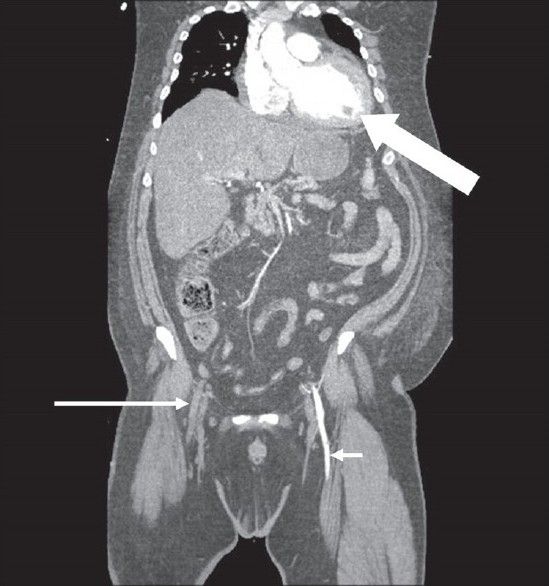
Coronal CTA image showing an occluded right external iliac/femoral artery (long arrow) when compared with the left side (short arrow). The left ventricular thrombus is visible (thick arrow)
